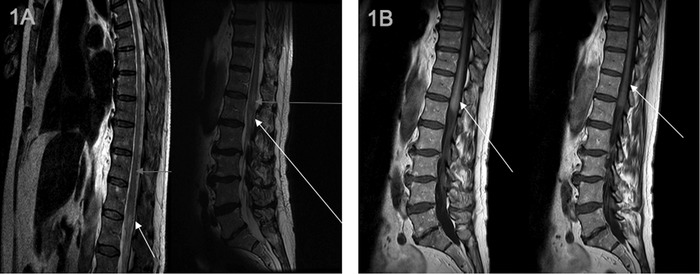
Sagittal magnetic resonance imaging with (A) T2 spin echo (TSE) sequence reveals hypersignal of the spinal cord from T6 to the terminal cone (arrows) and flow voids behind the spinal cord that are (B) enhanced with gadolinium injection. The hypersignal of the spinal cord on TSE sequence corresponds to chronic hypoxic congestive myelopathy attributable to venous hyperpressure hindering venous return and caused by an arteriovenous shunt in spinal cord vascularization. The arrows in views A and B correspond to congested small venous vessels that are hard to visualize because they are quite thin.
